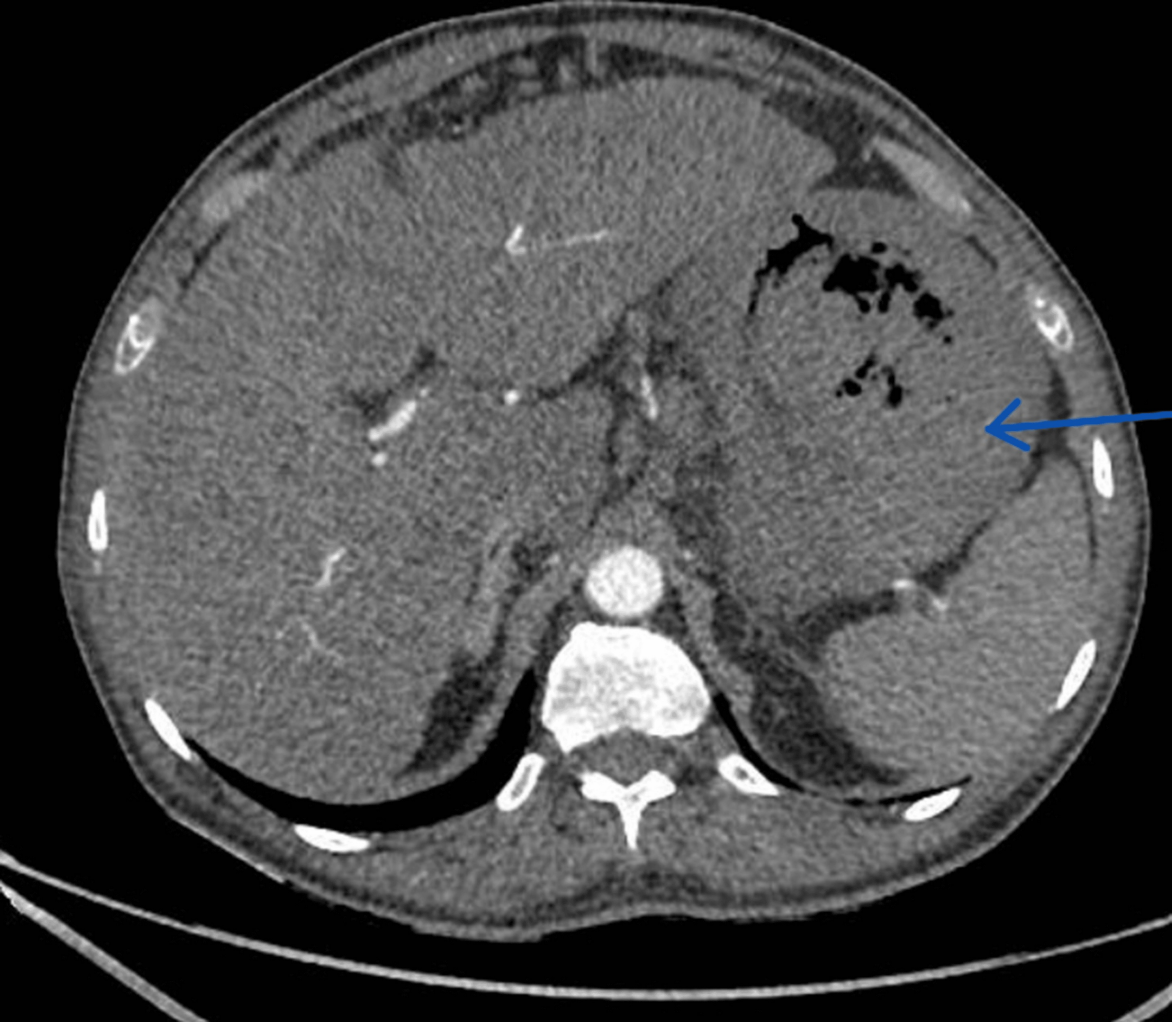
CT angiography of the abdomen. The blue arrow indicates the gastric wall is markedly thickened, and the flat planes along the lesser gastric curve show infiltration consistent with an inflammatory process or with arterial/venous ischaemia.
